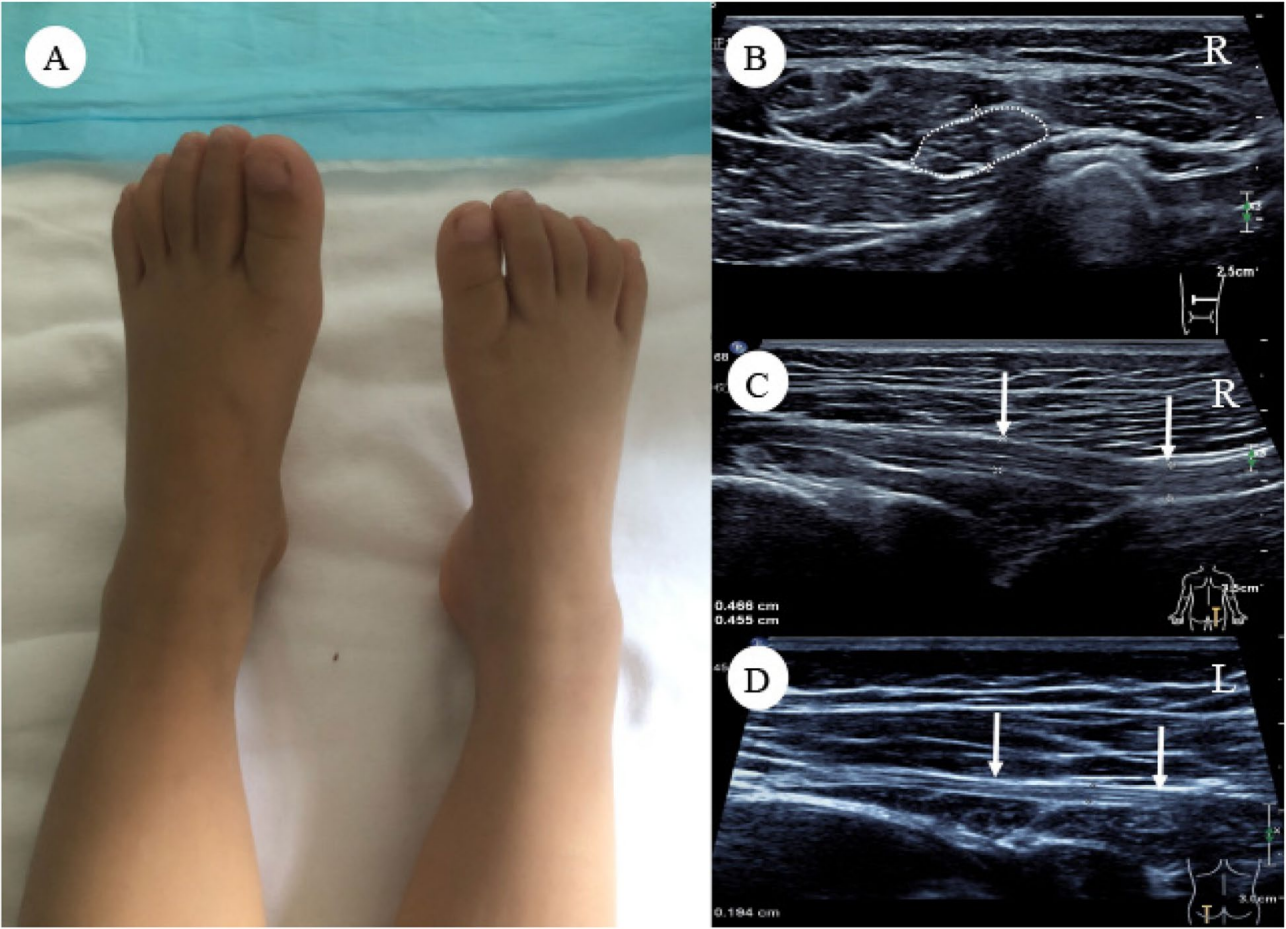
Images of patient #7. A 3-year-old girl with typical clinical manifestations of neuromuscular choristomas (NMC) of the sciatic nerve, including leg length discrepancy and calf muscles atrophy, and right foot shorter than the left foot (A). Ultrasound imaging demonstrated that the right sciatic nerve of the upper and middle thigh was hypoechoic and fusiformly enlarged. The cross-sectional area of the nerve was 0.68cm^2^ (B), and the diameter was 0.47 cm in the longitudinal section (C). Comparing with the other side, the size and echo intensity were normal in the left sciatic nerve (diameter 0.19 cm, cross-sectional area 0.23cm^2^) (D). Neither progression of NMC nor neuromuscular choristomas associated desmoid-type fibromatosis (NMC-DTF) was found during the 8-month follow-up
